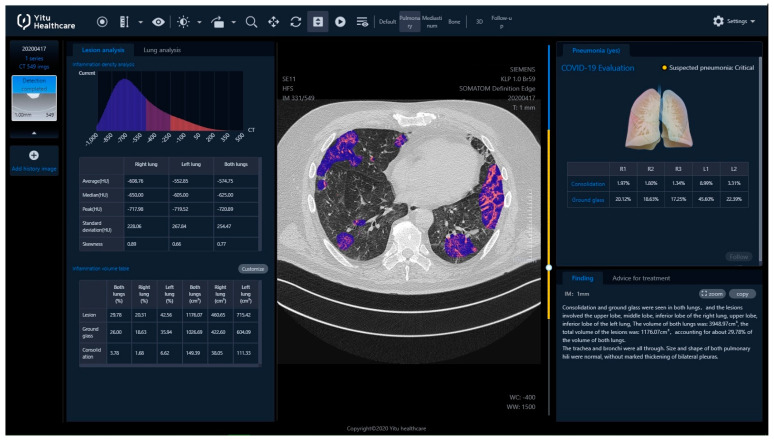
The final report from automatic assessment of HRCT images by the software, using artificial intelligence technology.
